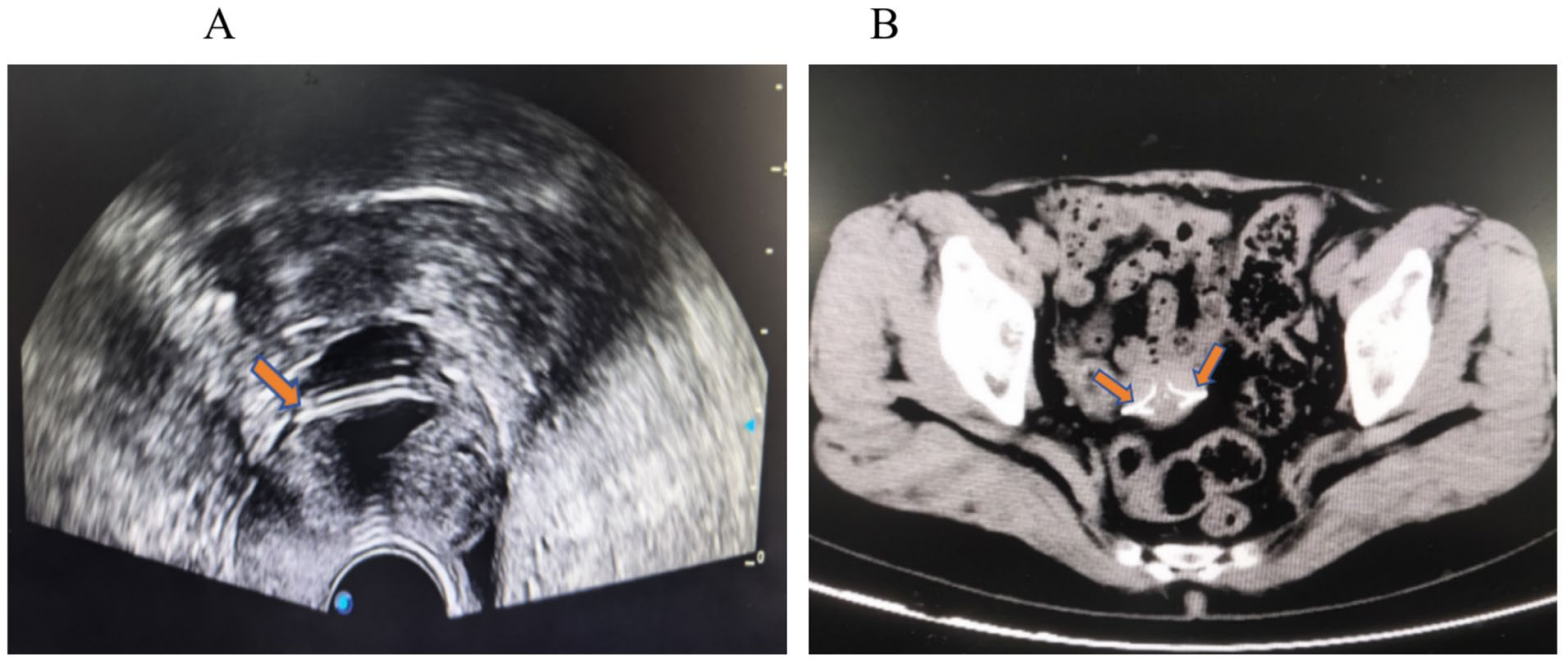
(A) Gynecological ultrasound: the right side of the IUD appears to reach the extraserosal aspect of the uterus. (B) Pelvic CT: the IUD is incarcerated, with edges protruding from the serosal surface of the uterus.
